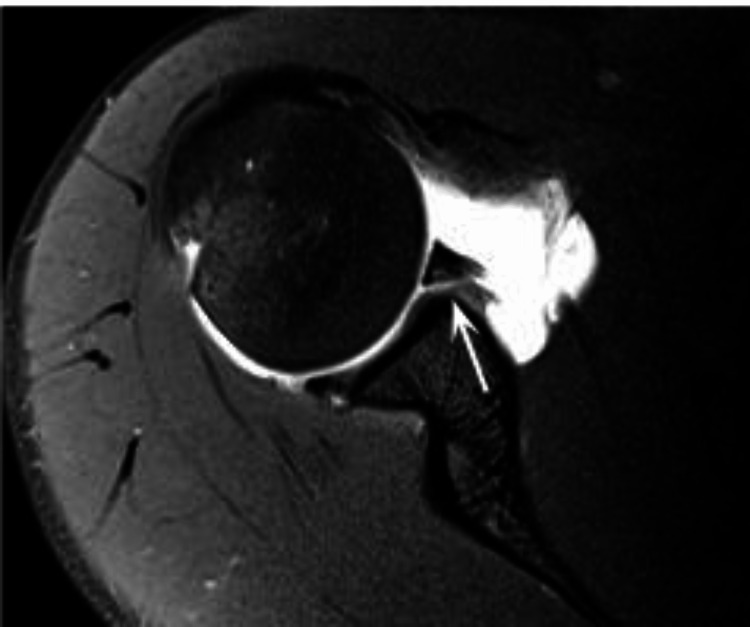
Axial fat-suppressed T1-weighted MRA image of the right shoulder The* *anteroinferior labrum has become detached from the glenoid (arrow) and is associated with a torn scapular periosteum - Bankart Lesion.
